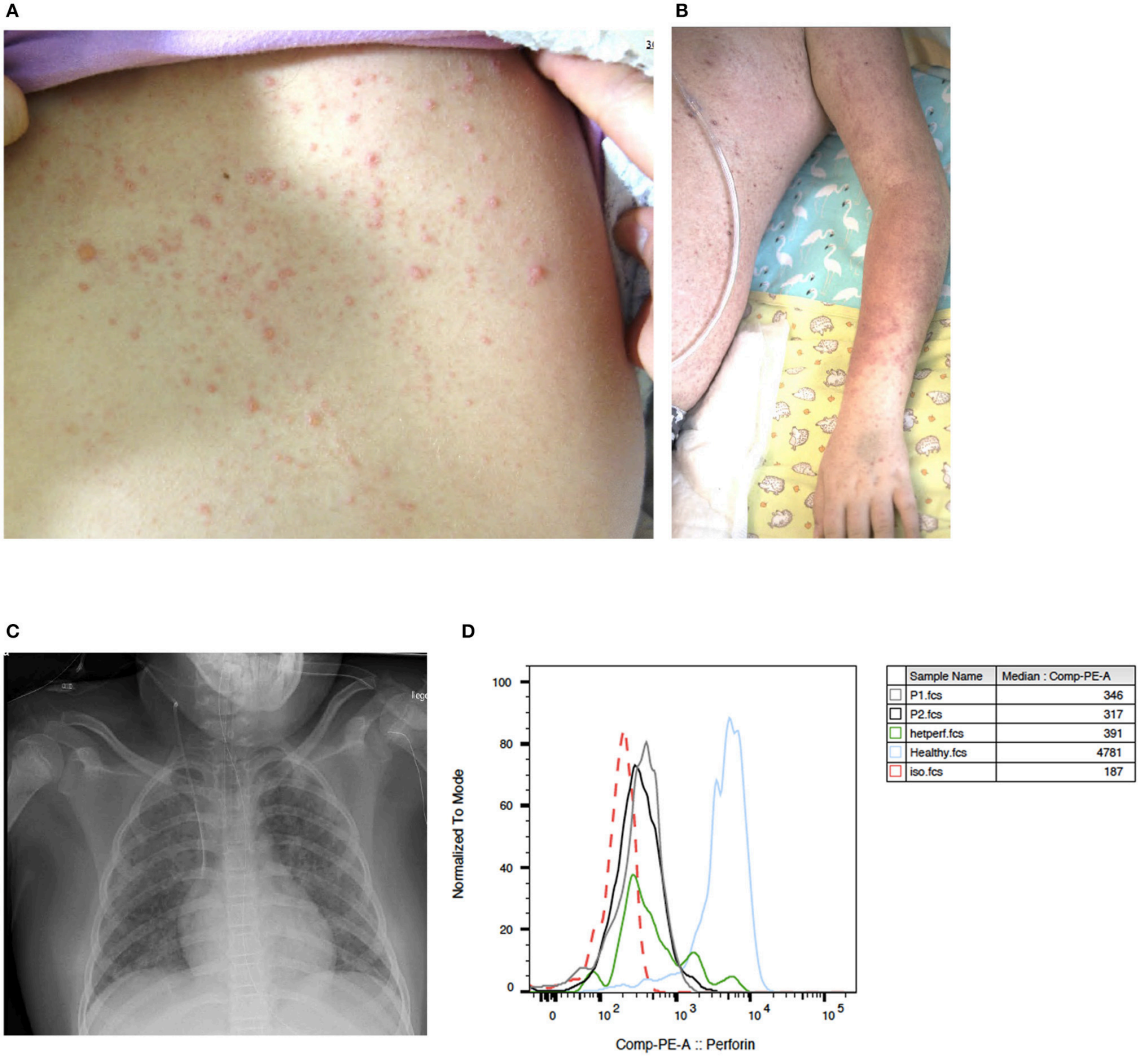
Clinical presentation of VZV disease in GATA2-haploinsufficient patient (A) An erytematous, vesicular skin rash on the back of patient 1 with GATA2-haploinsufficiency, 4 days after the onset of VZV disease. (B) Targetoid lesions on the left arm of the same patient, 7 days after the onset of illness. (C) A chest X-ray 8 days after the onset of illness, showing diffuse alveolar involvement with a miliary pattern of pulmonary opacities. (D) Histograms showing perforin expression in CD56+CD16+ lymphocytes from patient 1 (gray), patient 2 (black), an individual with heterozygous A91V PRF1 mutation (green), a healthy control (blue) and isotype control (red dotted line) as assessed by intracellular flow cytometry.
